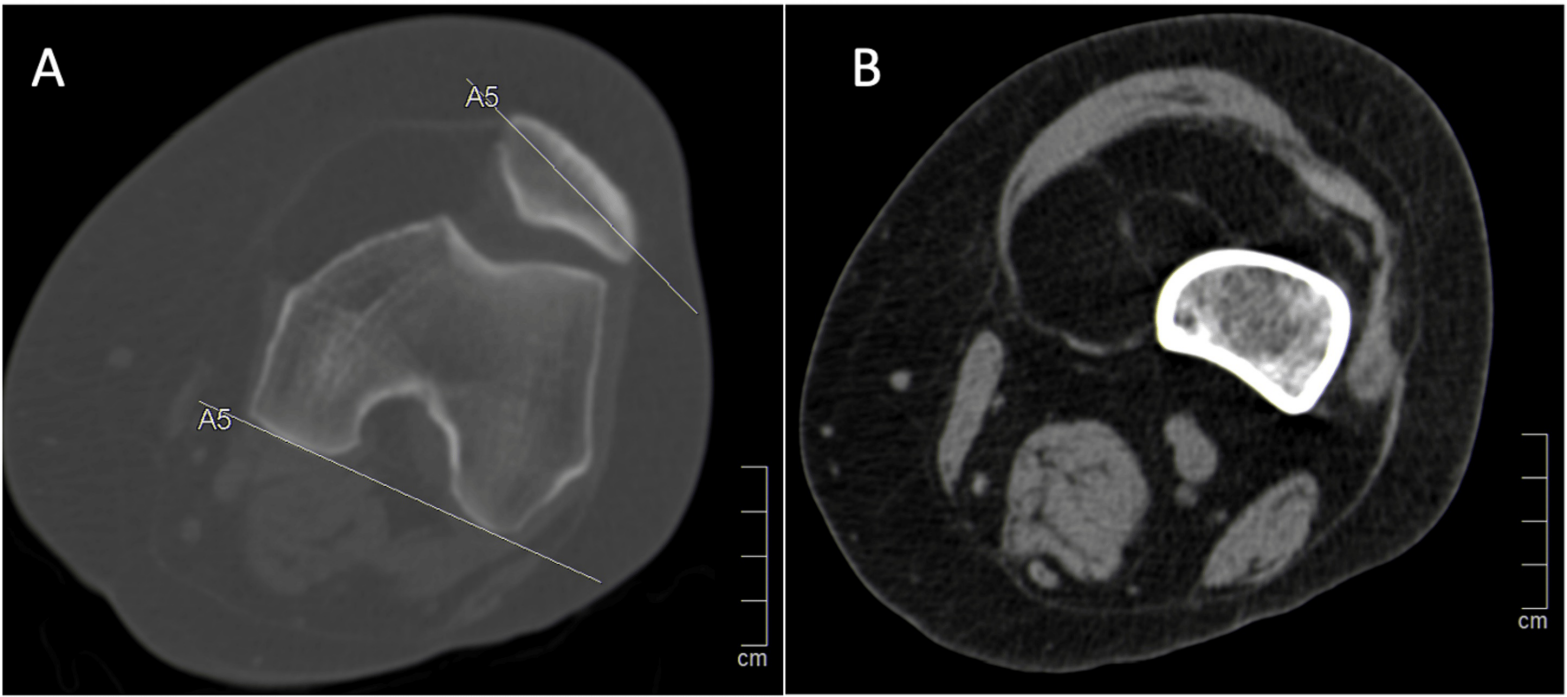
CT images of the left knee (A) Axial view showing a well-defined intra-articular lesion, with a slight patellar tilt of 22º due to mass effect. (B) Axial view showing the homogeneous fat attenuation mass with thin septations.
